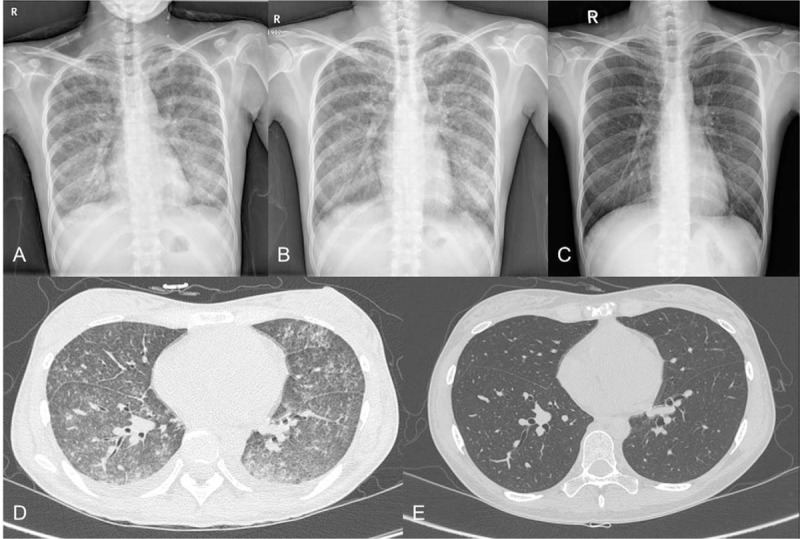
Imaging findings during deterioration and improvement of diffuse alveolar hemorrhage. A. Chest X-ray showing reticular opacity in the whole lung field following the development of respiratory distress. B. Chest X-ray showing no significant changes with plasma exchange alone. C. Chest X-ray showing marked improvement in diffuse lung infiltration after combination therapy with rituximab and plasma exchange. D. Chest CT revealing diffuse ground-glass opacity in both lung fields. E. Chest CT showing improvement in diffuse ground-glass opacity with interlobular septal thickening.
